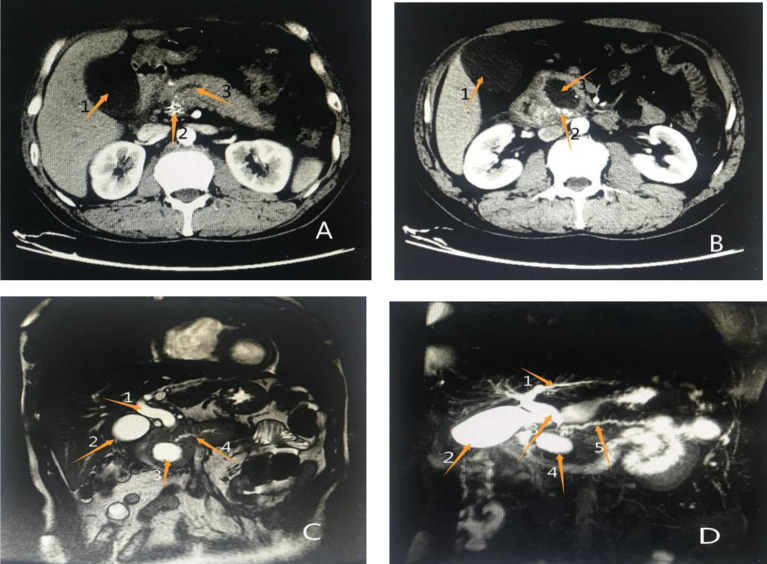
Results of previous imaging examinations at another hospital (A) (enhanced abdominal CT): Arrow 1 shows an enlarged gallbladder, arrow 2 shows patchy enhancement of the pancreatic head, and arrow 3 shows a significantly dilated main pancreatic duct. (B) Enhanced abdominal CT: Arrow 1 shows an enlarged gallbladder, arrow 2 shows patchy enhancement of the pancreatic head, suggestive of pancreatitis, and arrow 3 shows a rounded, low-density shadow of the pancreatic head, considered a pseudocyst. (C) Abdominal MRI: Arrow 1 shows a dilated common bile duct, arrow 2 shows an enlarged gallbladder, arrow 3 shows a pseudocyst of the pancreatic head, and arrow 4 shows full dilation of the main pancreatic duct. (D) Abdominal MRCP: Arrow 1 shows a dilated intrahepatic bile duct, arrow 2 shows an enlarged gallbladder, arrow 3 shows a dilated common bile duct, arrow 4 shows a pseudocyst of the pancreatic head, and arrow 5 shows a fully dilated main pancreaticduct.
